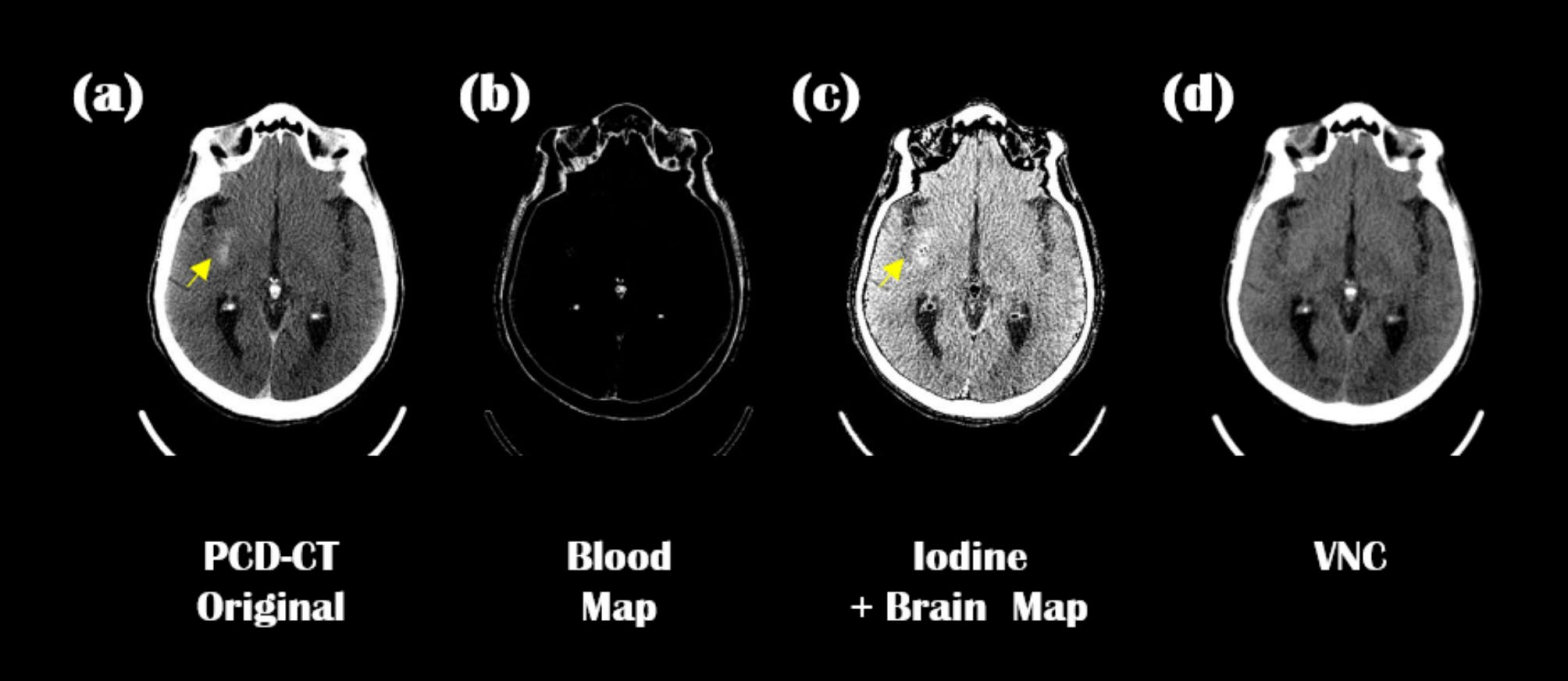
The hyperdense area on the PCD-CT image, and corresponding to material decomposition maps; PCD-CT original image (a), blood map (b); iodine combined with brain map (c); VNC (d).
